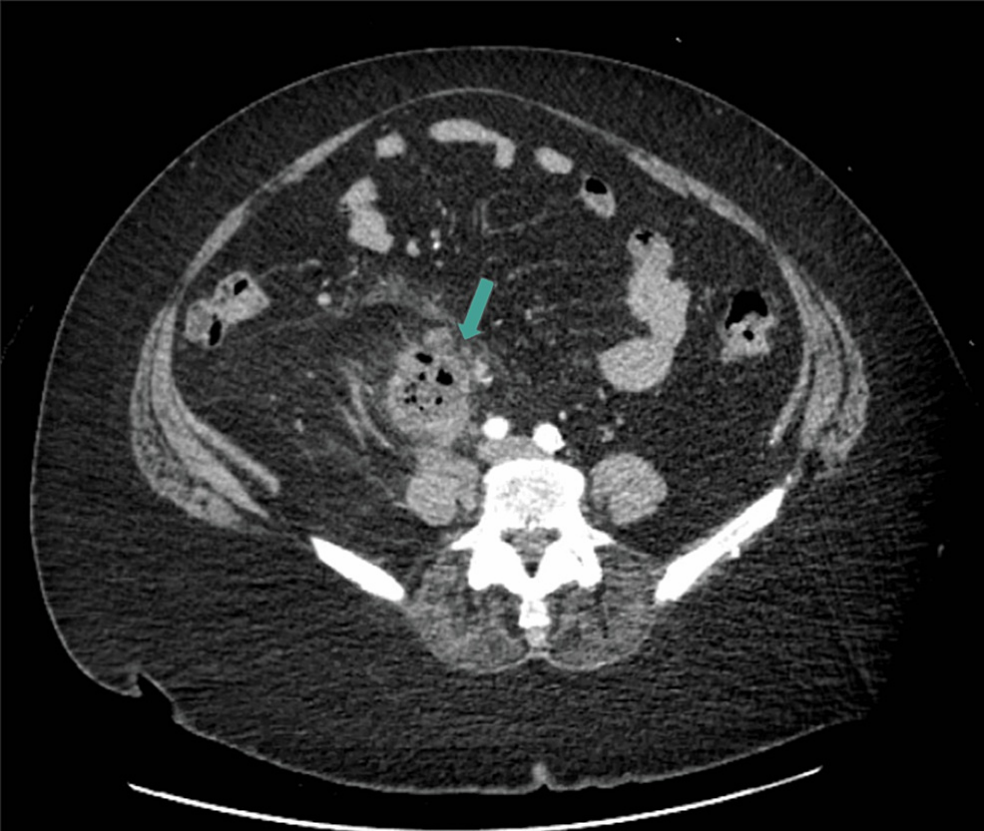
Cross-sectional CT transverse view demonstrating a gas- and fluid-filled peri-ureteral structure, consistent with abscess CT: computed tomography
